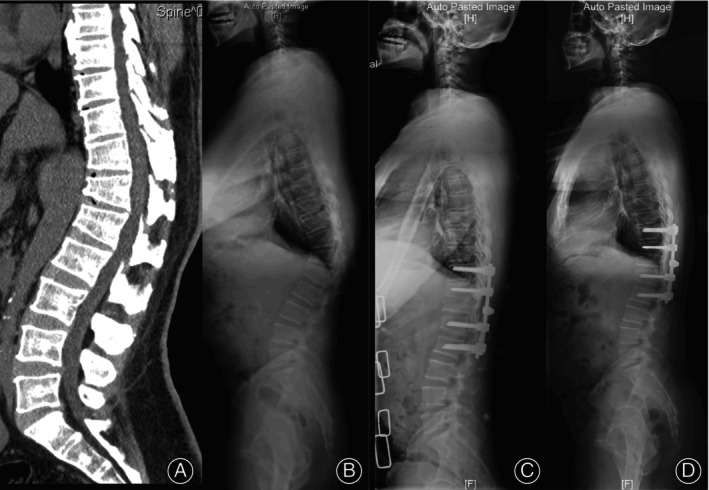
A 53‐year‐old man with degenerative thoracolumbar hyperkyphosis (DTH) arising from Scheuermann's kyphosis (SK). (A) Computed tomography showed wedging T11‐L2 vertebrae. (B) Lateral x‐ray showed global kyphosis (GK) and thoracolumbar kyphosis (TLK) were both 58.2°, sagittal stable vertebrae (SSV) located at L3. (C) Postoperative x‐ray showed fixation of two levels above and below the apex and modified grade 4 osteotomy, SSV moved cranially to L2, GK and TLK decreased to 21.9°. (D) Solid bony fusion could be seen without junctional complications at 2‐year follow‐up, GK and TLK maintained.
